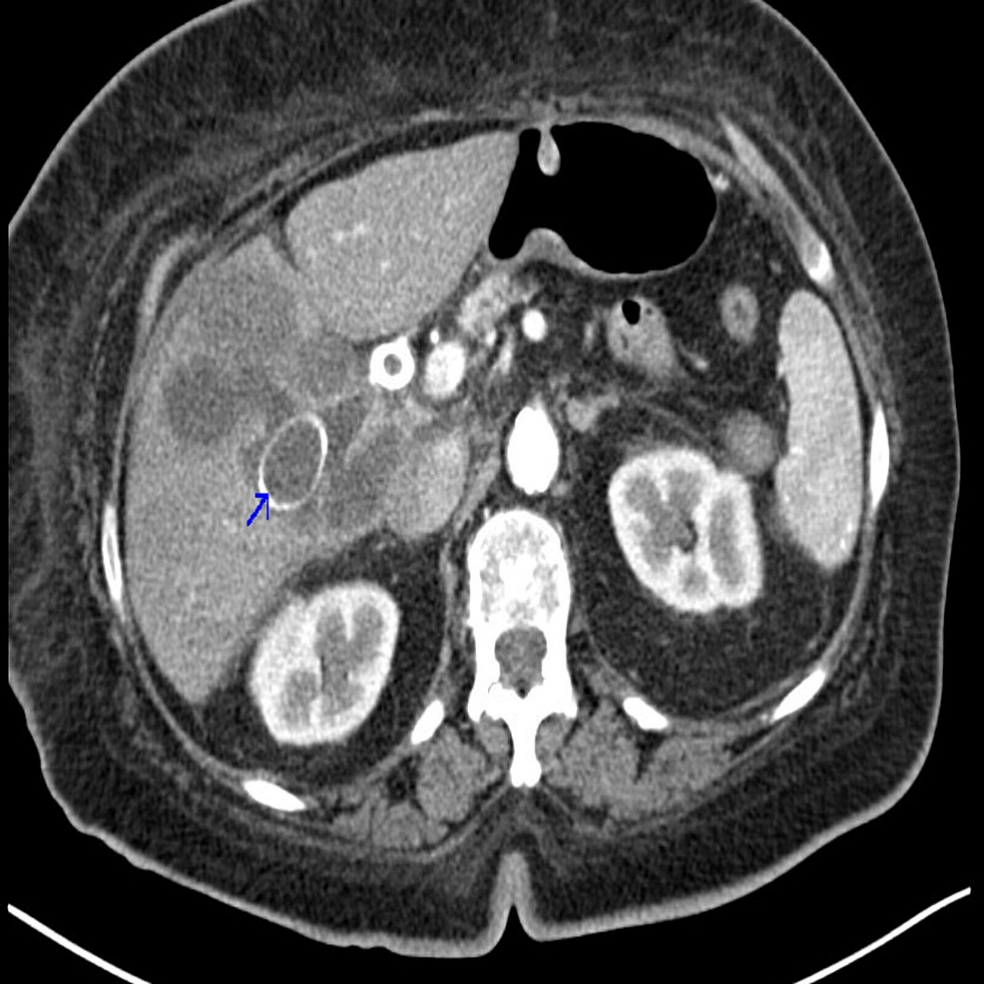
CT scan of the abdomen showing a large necrotic appearing mass.
